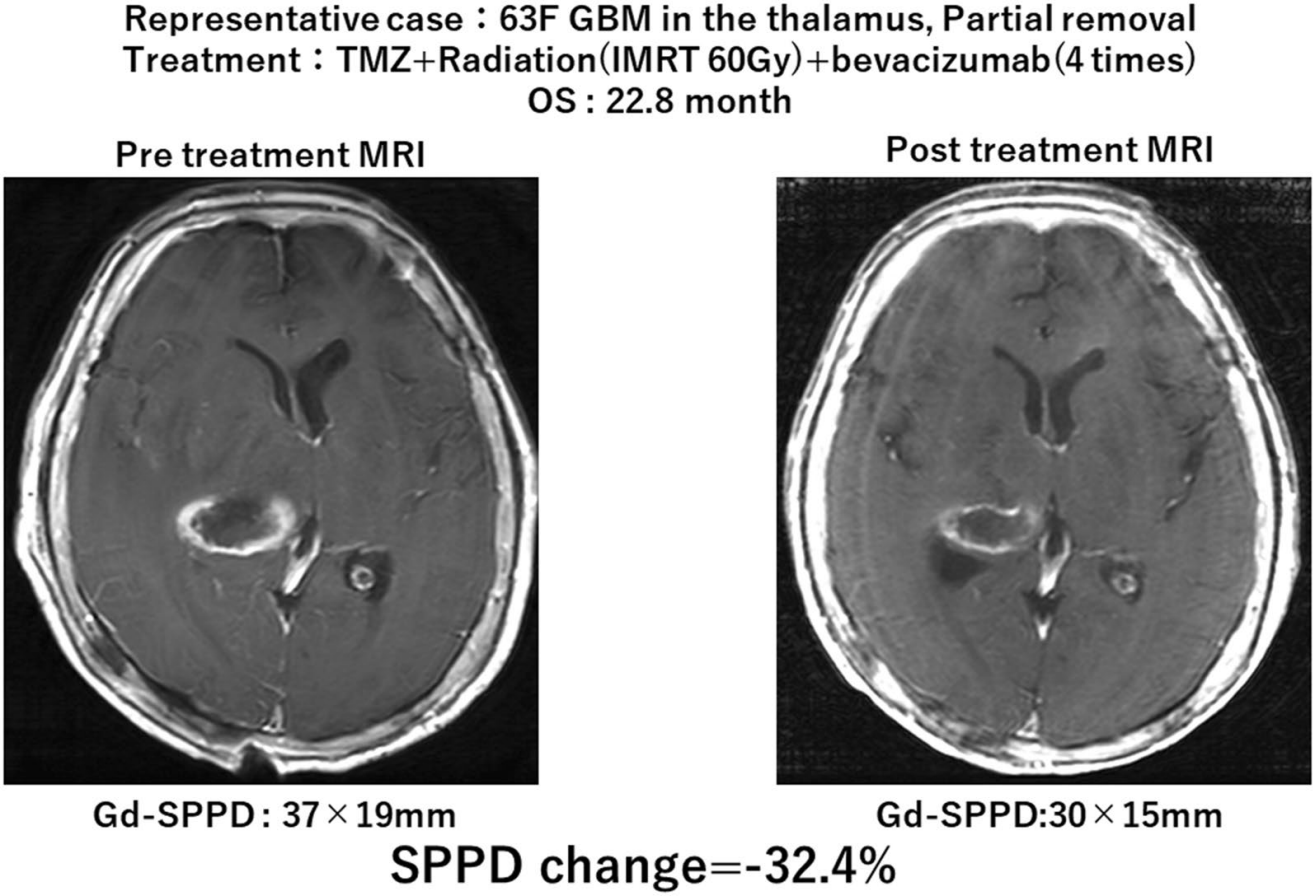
A representative case of discrepant result: 63-year-old female. We performed partial removal of the tumor. The patient was treated with temozolomide (TMZ; 75 mg/m^2^/day) and radiotherapy (Intensity Modulated RT60 Gy) and bevacizumab (four times in total). On the pre-RT magnetic resonance imaging (MRI), enhancing lesion and the sum of the product of the perpendicular diameter (Gd-SPPD) was 37 × 19 mm. After RT, GdT1WI showed a reduction in interpretation by the neuroradiologist. However, Gd-SPPD was 30 × 15 mm and the change in SPPD was 32.4%, which was determined as SD based on the Response Assessment in Neuro-Oncology (RANO) criteria. OS at 22.8 months. *GBM* glioblastoma; *RT* radiotherapy; *SD* stable disease; *TMZ* temozolomide
